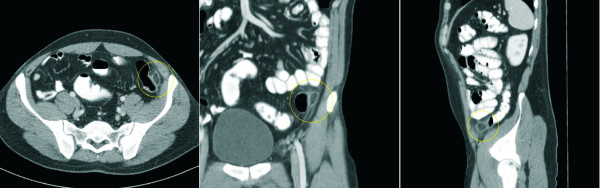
Abdominal CT scan demonstrating the horizontal, coronal and sagittal section of a patient with EA (circle).
